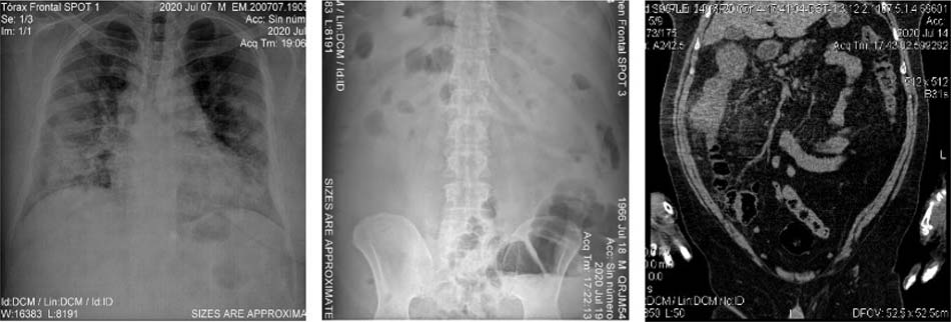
Imaging studies; (A) patient’s chest X-ray with bilobular diffuse pulmonary and alveolar infiltrate along with multiple opacities are present; (B) patient’s abdominal X-ray with dilated small intestine and colon with an occlusion located at the hepatic angle; edema and abdominal air fluid levels are noted; (C) abdominal CT scan showing engrossment of the ascending colonic wall at the hepatic angle with inflammation of pericolic adipose tissue; no free air or liquids are appreciated, suggesting a colonic occlusion.
